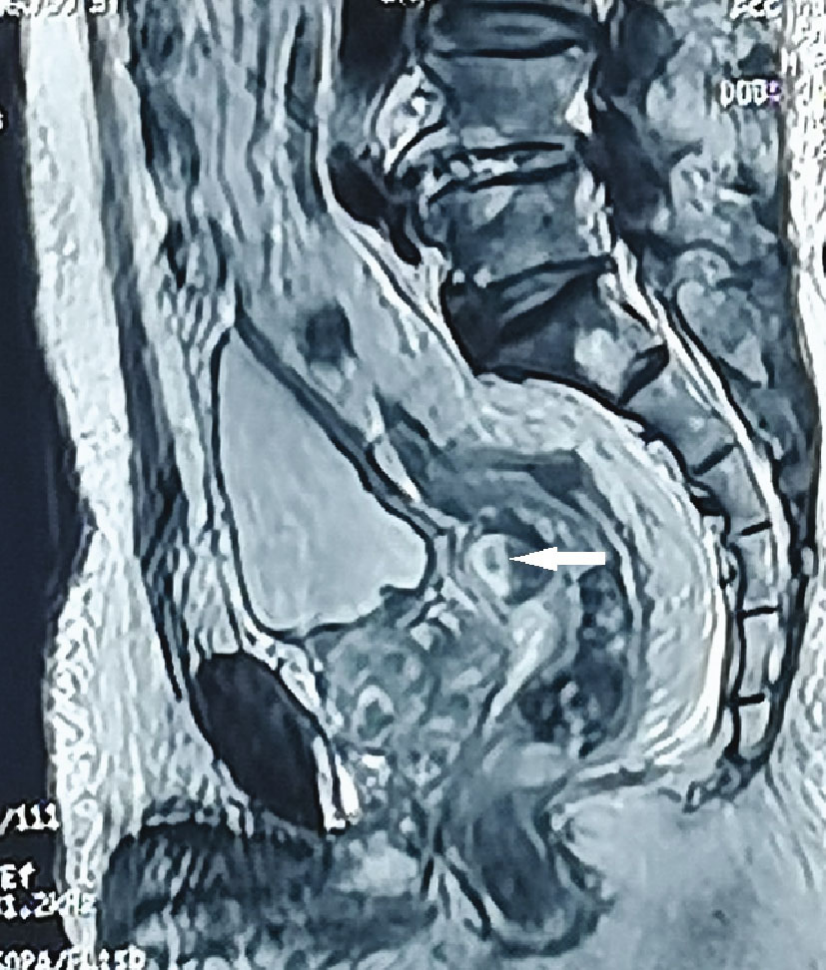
Pelvic abscess basically disappears on follow-up MRI (white arrow) after aspiration in 2015.
